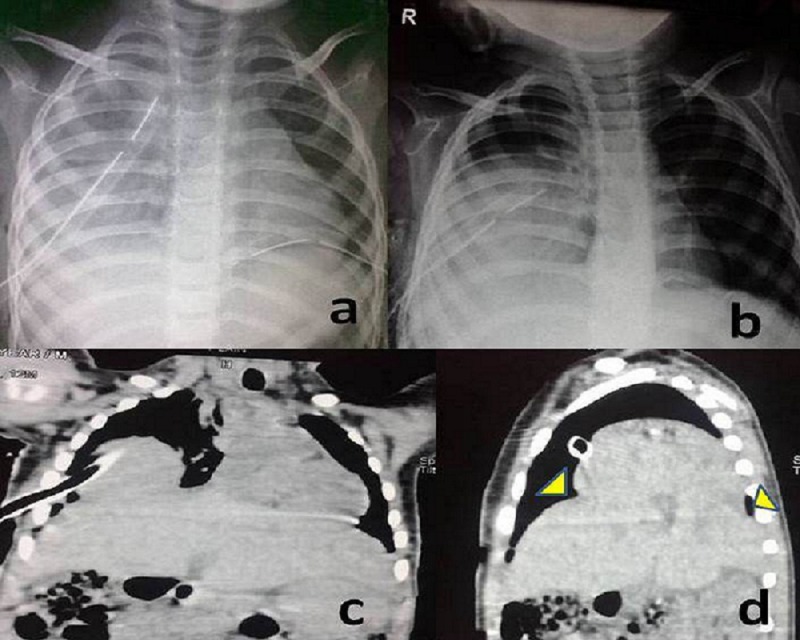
Figure 1: Figure 1: (a) Radiograph - immediately after inserting chest tubes. (b) Persistent radio-opacity in right lower zones after a week of chest tube insertion. (c) Coronal section of CT thorax showing hepatothorax. (d) Sagittal section of CT thorax showing margins of diaphragm around hepatothorax (Arrow heads)
